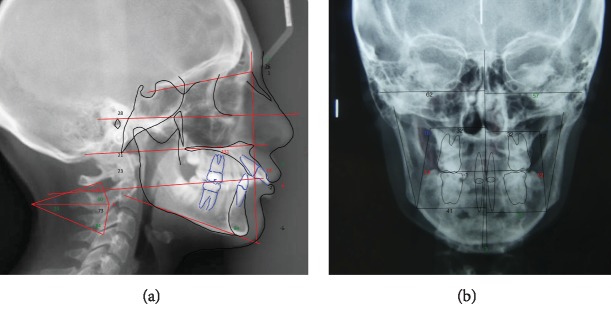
Cephalometric tracing (T0): (a) cephalometric evaluation of the lateral radiograph of the skull and (b) cephalometric evaluation of the posteroanterior radiograph of the skull; T0=pretreatment.
